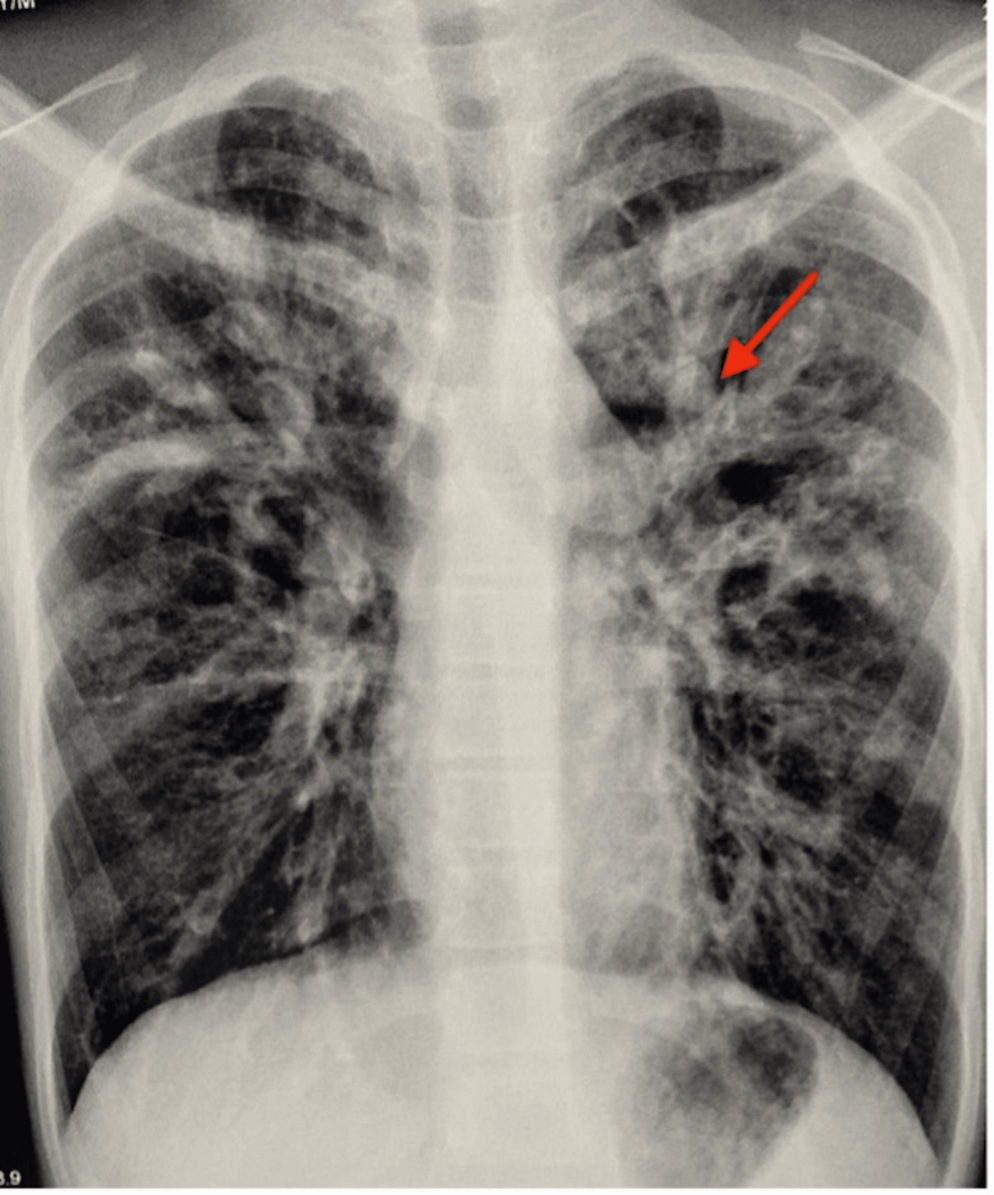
Chest X-ray showing gloved finger appearance Red arrow: gloved finger appearence
